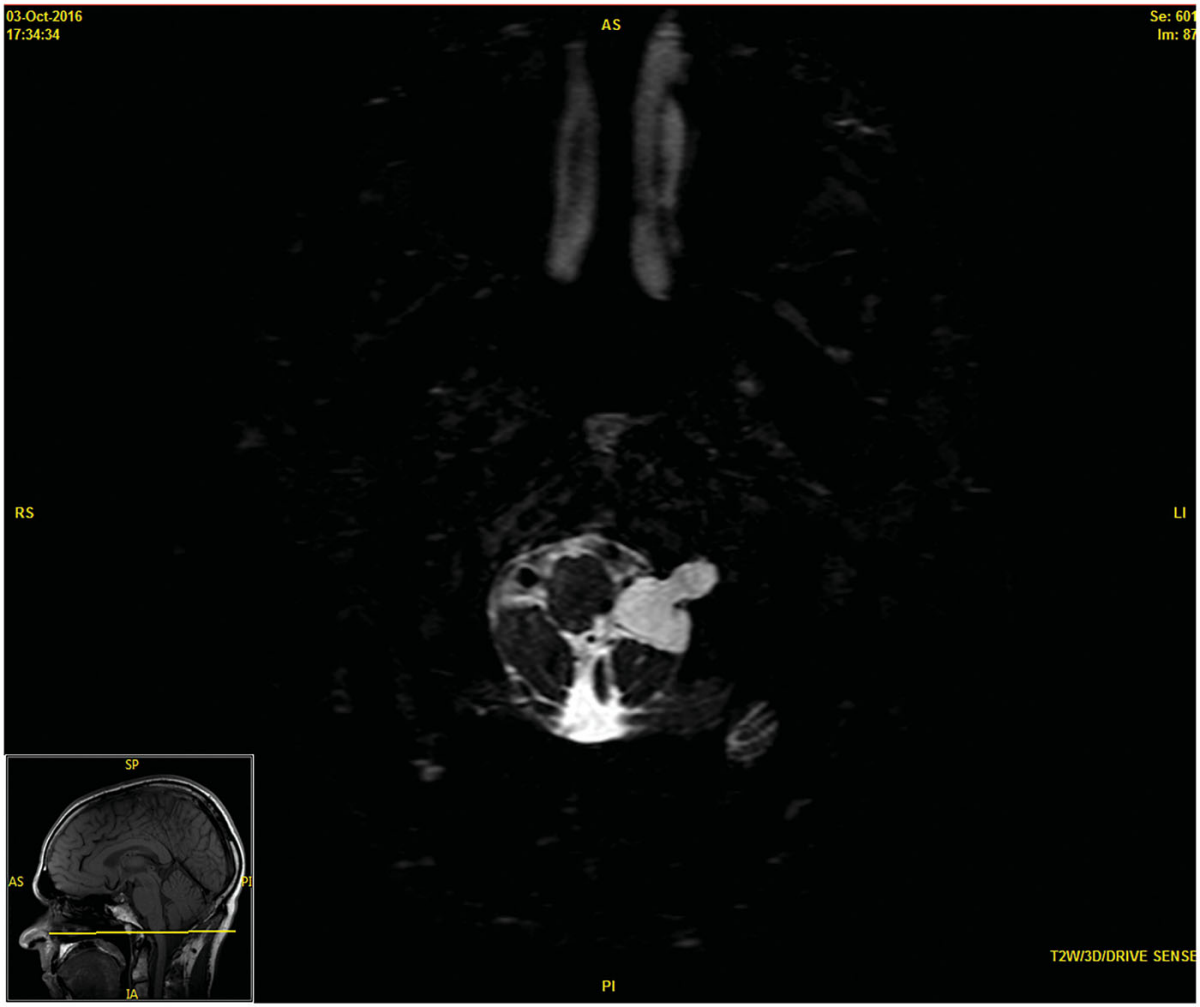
Follow-up T2 weighted 3D magnetic resonance image (MRI) 12 months after initial presentation demonstrating compression at the level of the brainstem.
